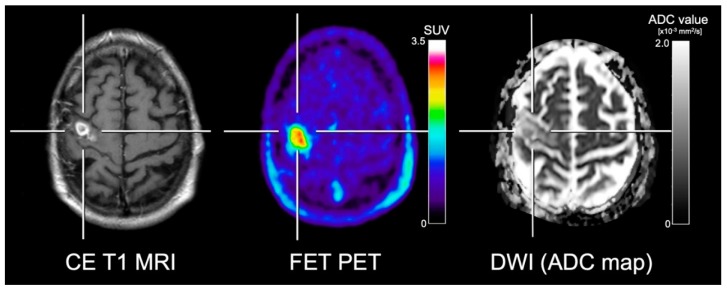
Contrast-enhanced (CE) T1-weighted MRI, FET PET and the apparent diffusion coefficient (ADC) map obtained from DWI in a patient with neuropathologically confirmed glioblastoma. In contrast to the ADC map, FET PET shows the metabolically active tumor tissue with high contrast. Furthermore, the ADC map shows slightly increased diffusivity in various subregions, erroneously indicating treatment-related changes.
